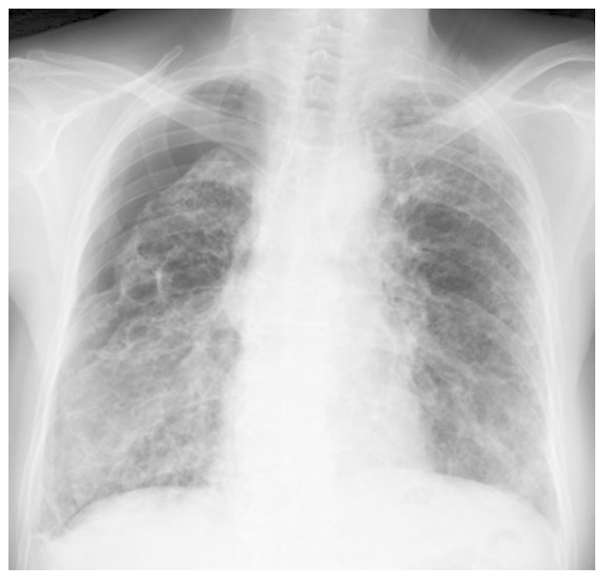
Chest radiograph reveals right lung pneumothorax, and reticulonodular opacities predominant in the bilateral lower lungs.
